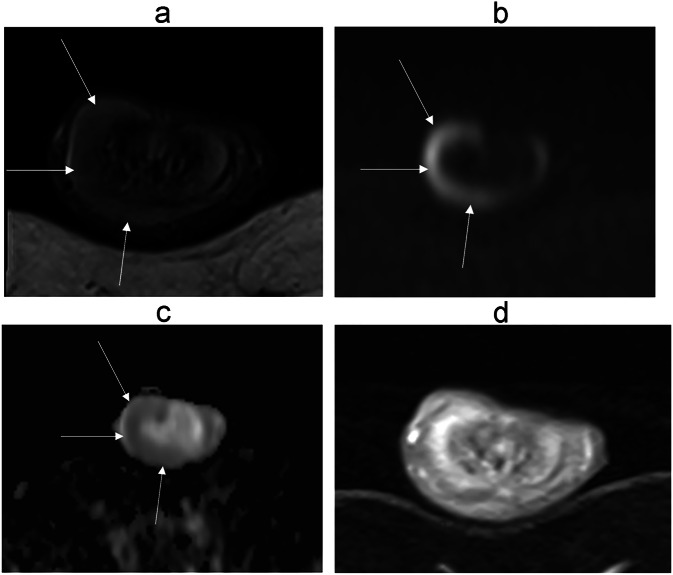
Sixty-seven-year-old patient, consulting in a context of phimosis and penile bleeding. On clinical examination, the lesion is classified as cT2. a Axial T2-W MR images, show an extensive tumor with involvement of the corpus spongiosum, predominantly on the right lateral side. b Axial DWI and (c) ADC map show intense diffusion restriction. The enhanced sequences (d) do not provide any further information here. Patient underwent distal partial amputation showing a squamous carcinoma of the glans, grade 2, infiltrating the corpus spongiosum without infiltration of the urethra. Stage pT2N0
